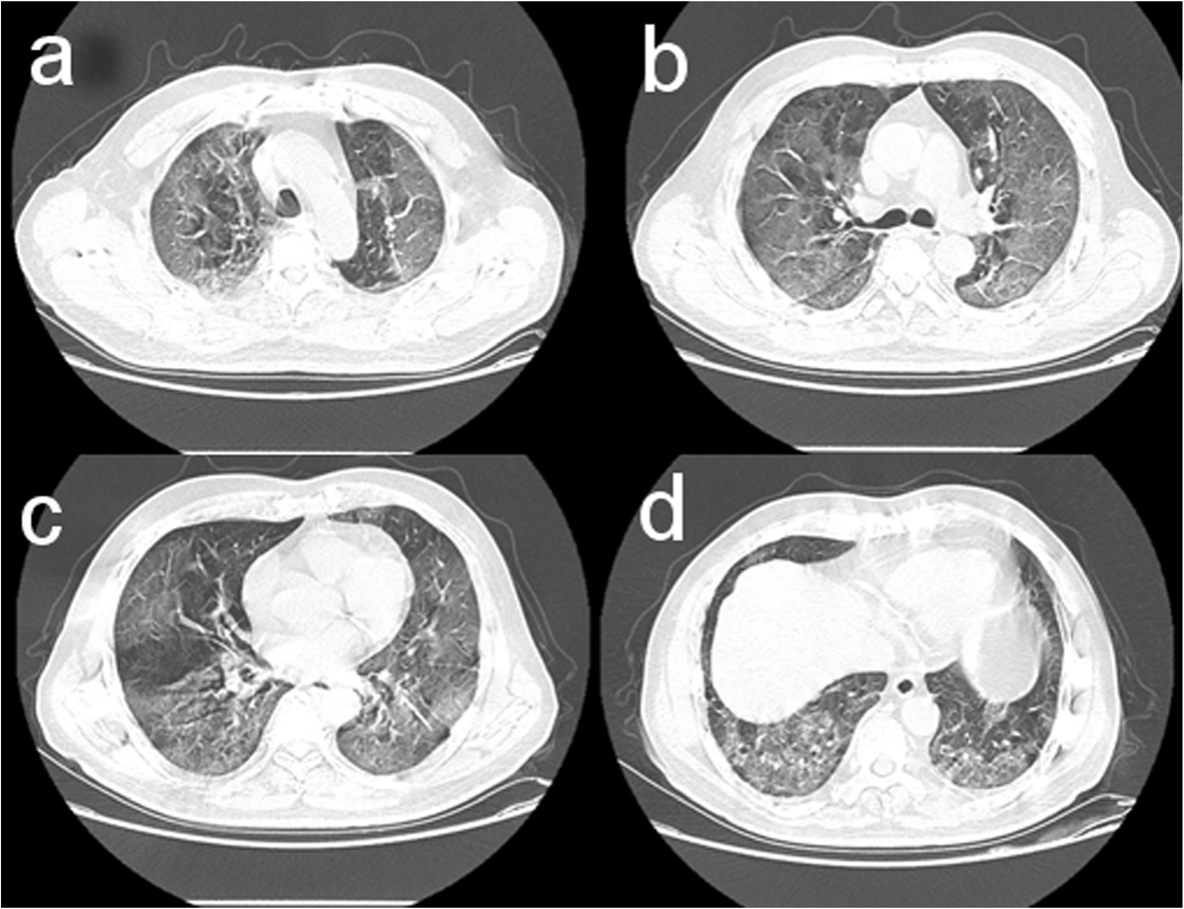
Chest computed tomographic imaging of the lungs performed on February 26, 2020, showed ground-glass opacity in the bilateral lungs on day 10 after symptom onset
